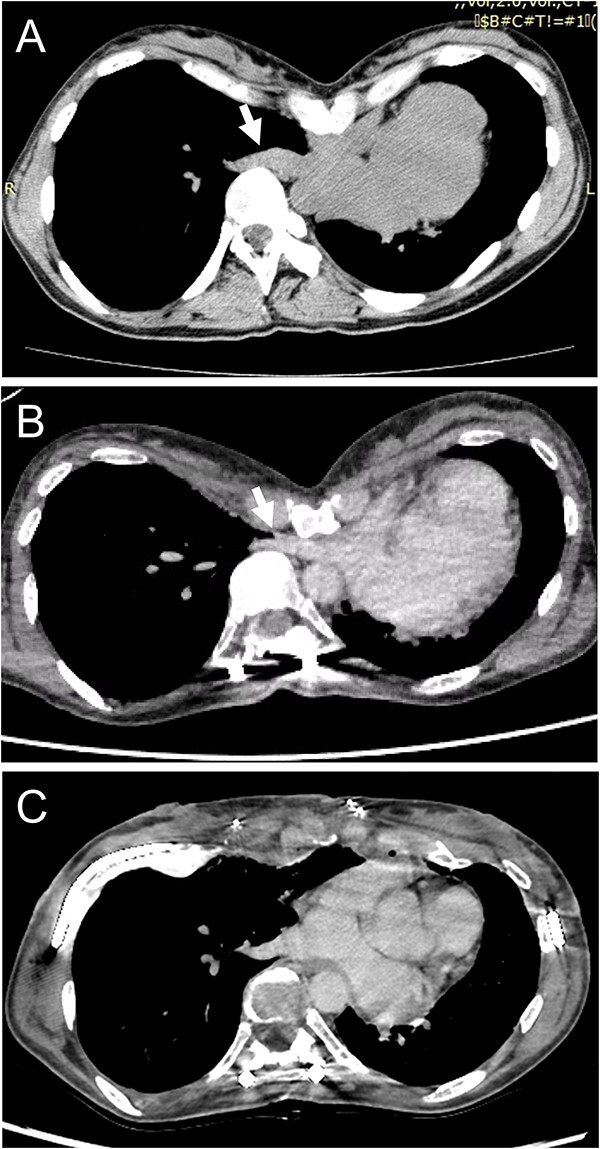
(A) Chest CT shows no compression of the right inferior pulmonary vein (IPV) before scoliosis repair (arrow). (B) Severe right IPV stenosis is induced by posterior spinal fusion (arrow). (C) Postoperative chest CT indicates that right IPV stenosis is released by elevation of the depressed sternum.
